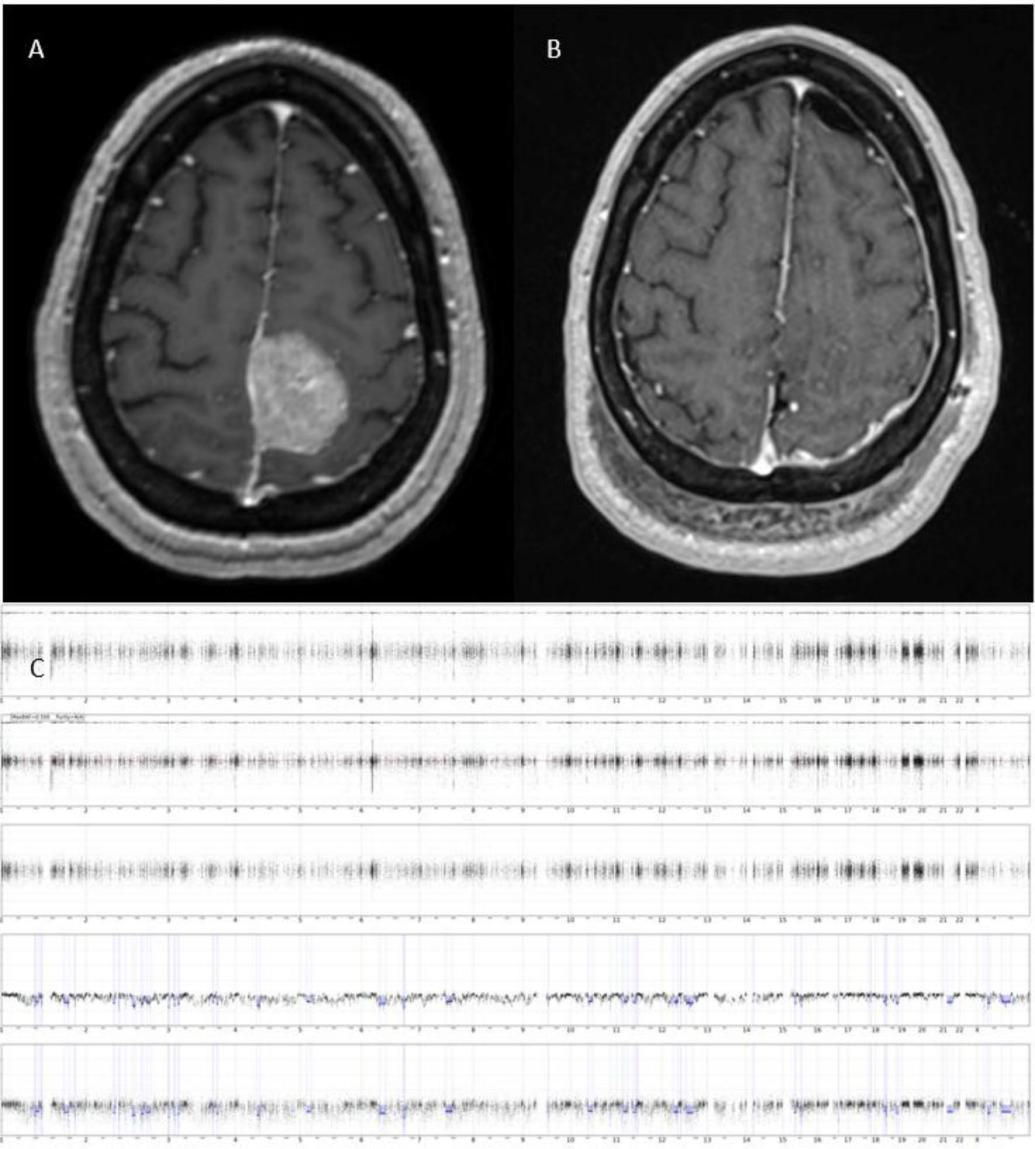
T1 MRI with contrast demonstrating an asymptomatic parafalcine meningioma before (A) and after (B) gross total resection. Intraoperative pathology confirmed a WHO grade 2 meningioma. However, further genetic profiling of the tumor demonstrated a more benign genomic signature suggestive of a lower-grade meningioma, with no copy number variations (CNV) demonstrated on CNV plot (C). The postoperative course of this patient was uncomplicated; following adjuvant radiation therapy, the patient was followed closely with surveillance imaging
